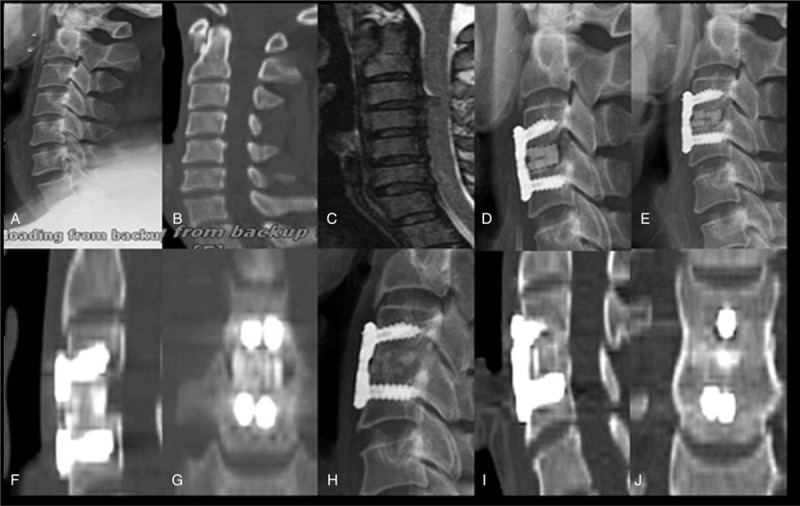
The graph shows a case using BioCage for ACDF. The bone fusion can be achieved at 3 months postoperative. At the last of follow-up, the cage was replaced completely by new bone formation. A 46-year-old man with cervical spondylotic myelopathy. (A–C) preoperative lateral X-ray films, preoperative computed tomography (CT) and magnetic resonance imaging (MRI); (D) lateral X-ray films 3 days after operation; (E-G) X-ray 3 months after operation and CT 3 months after operation; (H-J) X-ray 46 months after operation and CT 46 months after operation.
